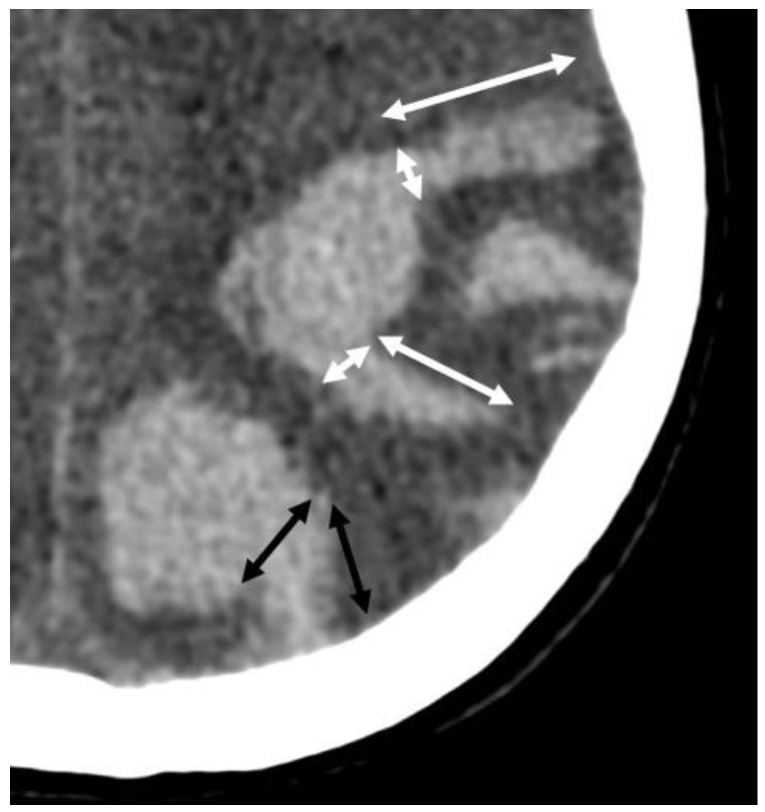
Finger-like projections on non-enhanced CT (slice thickness 1 mm, 100 kV, 394 mAs). The length of the projection exceeds its width at the base where it emerges from the main hemorrhage (white double arrows). The lobulation of a hemorrhage with the width of the base approximately the same as its length (black double arrow).
